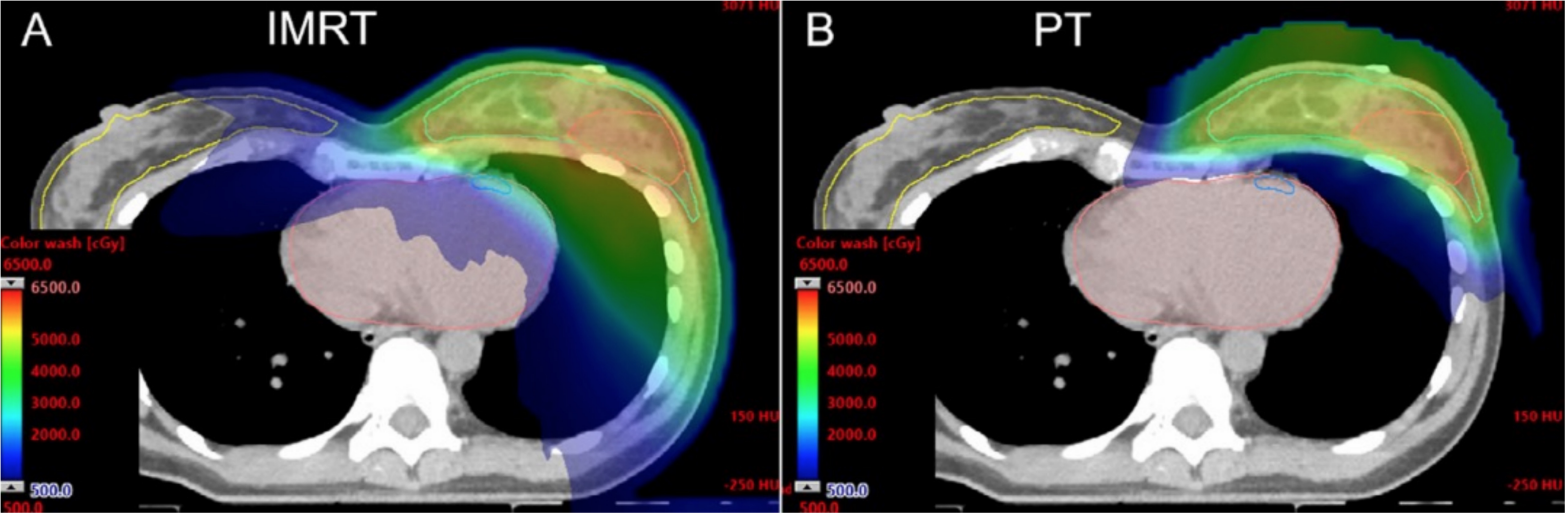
Computed tomography (CT) dose color wash from an IMRT plan (A) and pencil-beam scanning PT plan (B) [Mutter et al. (13)].
